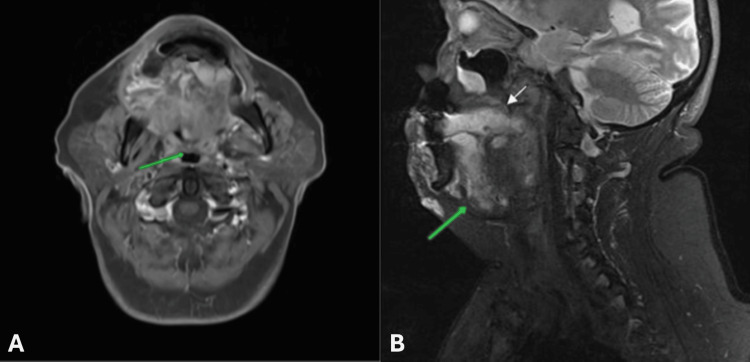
Head and neck magnetic resonance imaging (MRI) findings of a large lobulated mass extending across the entirety lingual dorsum (presulcal and postsulcal) with substantial inhomogeneous enhancement following intravenous (IV) contrast administration (A): The mass was hypointense on axial T1 fluid-attenuated inversion recovery (FLAIR) sequences, and oropharyngeal airway narrowing was displayed (green arrow). (B): Sagittal T2 sequences revealed a hyperintense lesion with multiple small hypointense foci compatible with flow voids, abutting the palatinum cranially (white arrow) and the left of the lingual dorsum caudally (green arrow).
